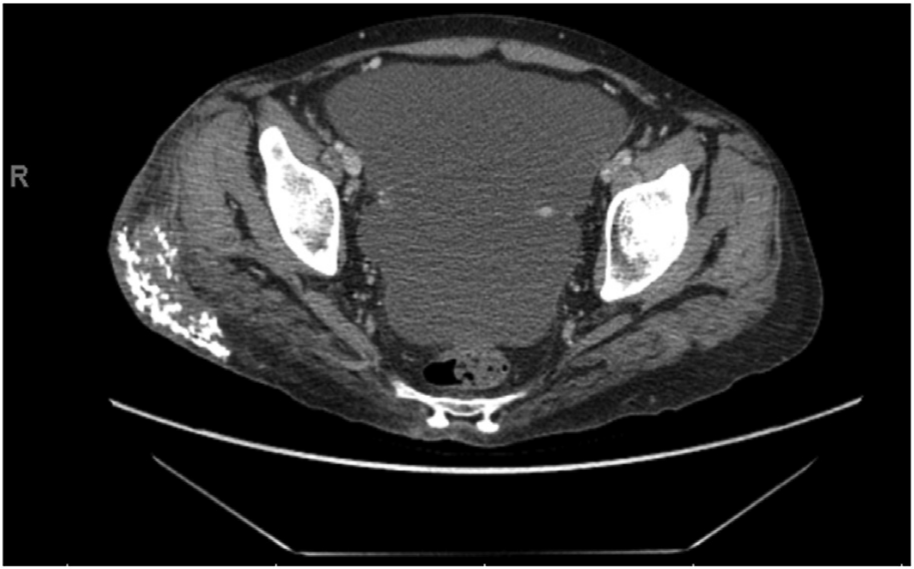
Computed tomography scan of patient’s pelvis obtained shortly after biopsy shown in Fig 1 with calcification in the *right* lateral thigh and gluteal subcutaneous fat. No underlying osseous destruction was noted.
